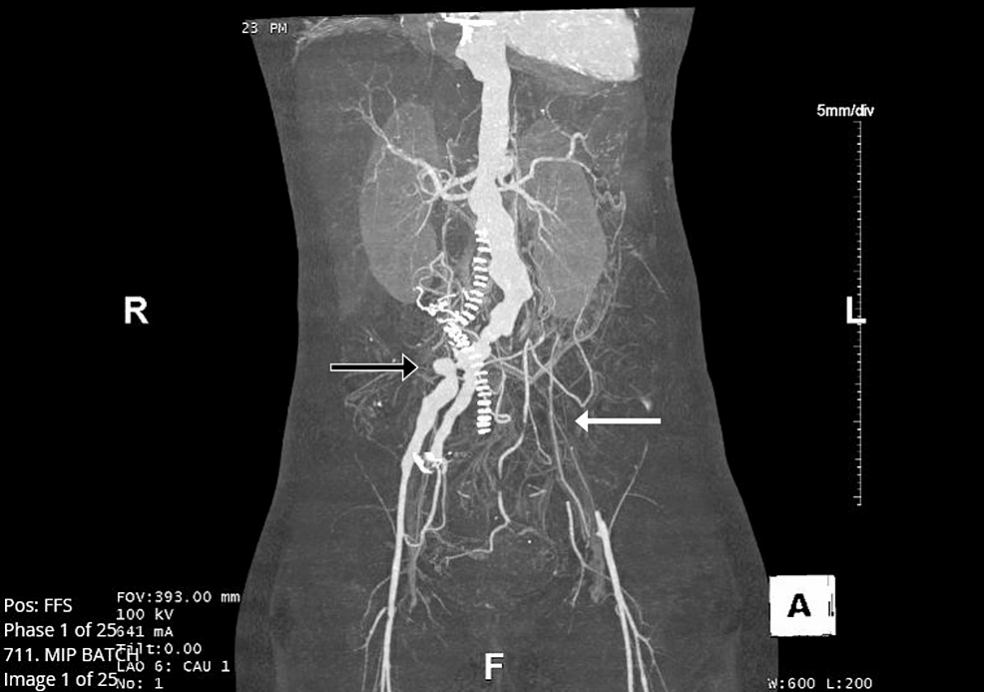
CT angiogram of the abdomen and pelvis Angiogram of the abdomen and pelvis showing a saccular aneurysm at the bifurcation of the right common iliac artery (black arrow) with focal high-grade stenosis at the right external iliac artery and proximal occlusion of the right superficial femoral artery. An occlusive thrombus is visualized in the left common iliac artery, left internal iliac artery, and left external iliac artery (white arrow).
